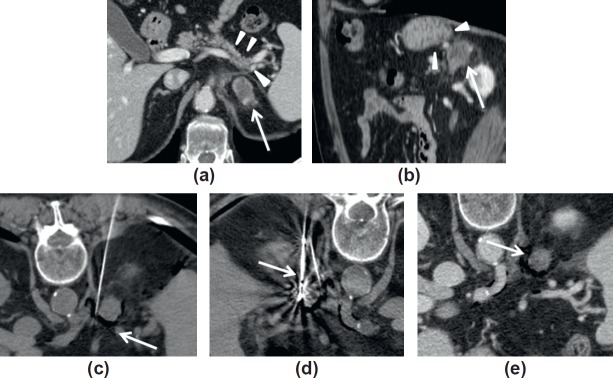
Case of an adrenal metastasis from lung cancer treated with percutaneous microwave ablation. (a,b). Preoperative CT demonstrated a 31 mm right adrenal metastasis (arrow) that was located in close proximity with critical structures such as pancreas (arrowheads in a) or stomach (arrowheads in b). (c). A small caliber needle was placed medially to the adrenal gland and CO_2_ injected in order to displace the lesion from surrounding critical structures. (d). A microwave antenna was inserted into the adrenal metastasis (arrow) and ablation was performed. (e). Final result 24 hours after ablation showing complete devascularisation of the lesion (arrow).
